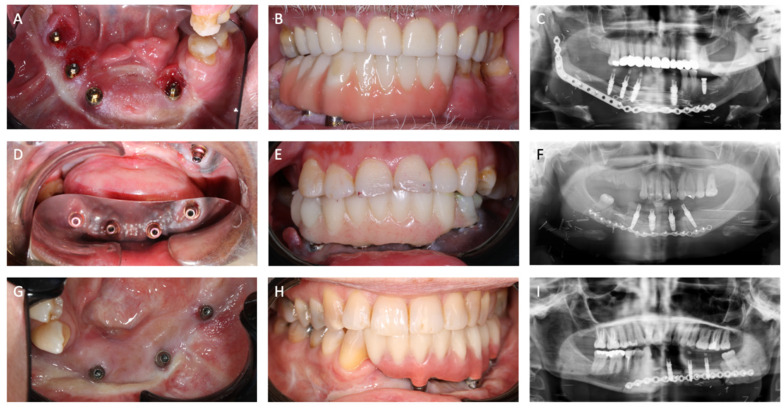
Clinical and radiographical end result of all 3 cases. (A–C): case 1, (D–F): case 2, (G–I): case 3.
